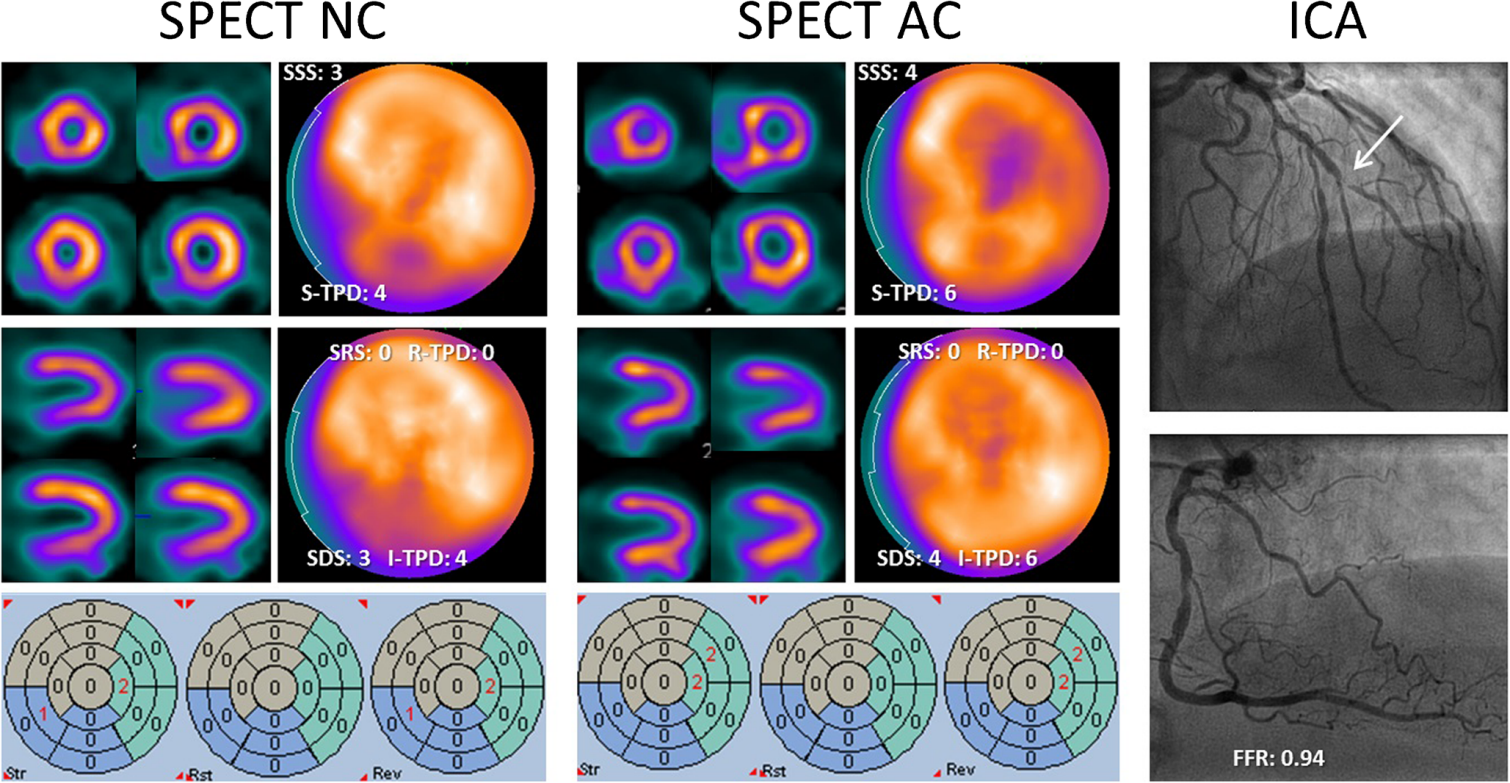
Representative SPECT images with and without AC, and invasive coronary angiography images of an 80-year-old male with typical angina. The left panel shows stress (upper row) and rest (lower row) images without AC. Only subtle perfusion reversibility can be observed in the anterior territory, whereas a fixed defect might be identified visually in the inferior territory. Automated grading revealed rather low scoring values, which were nonsignificant except for SDS and I-TPD. SPECT images with AC in the center panel display a slightly different perfusion pattern with more pronounced reversibility in the anterolateral segments, whereas the inferior wall is corrected into normal perfusion. Automated grading now clearly indicates ischemia in the anterior region only, instead of possible ischemia anterior and inferior. A sub-totally occluded diagonal branch but non-significant stenosis in the RCA on angiographic images (right panel) confirm the SPECT findings. AC = attenuation correction; FFR = fractional flow reserve; I-TPD = ischemic total perfusion deficit; NC = non-corrected; SDS = summed difference score; SRS = summed rest score; SSS = summed stress score; R-TPD = rest total perfusion deficit; S-TPD = stress total perfusion deficit
